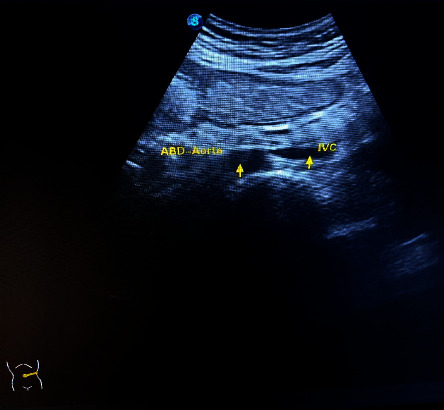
Ultrasound of periumbilical and epigastric region showing anatomical reversal of abdominal aorta and inferior vena cava (IVC).
